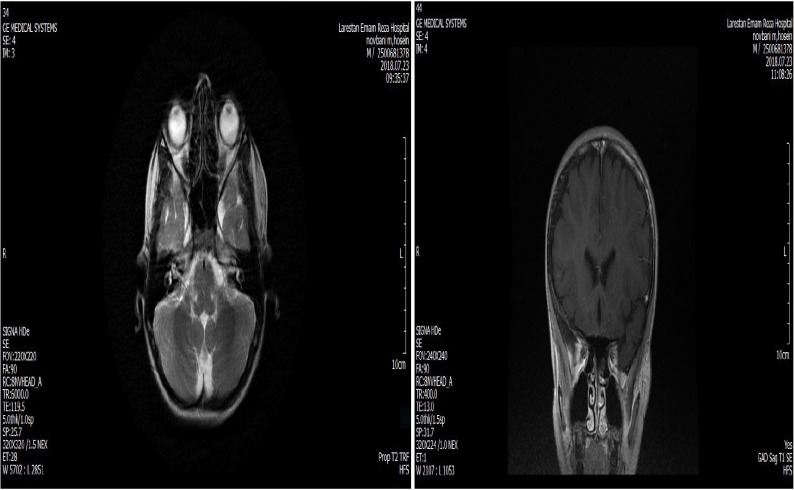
MRI revealed an ill-defined soft tissue mass
